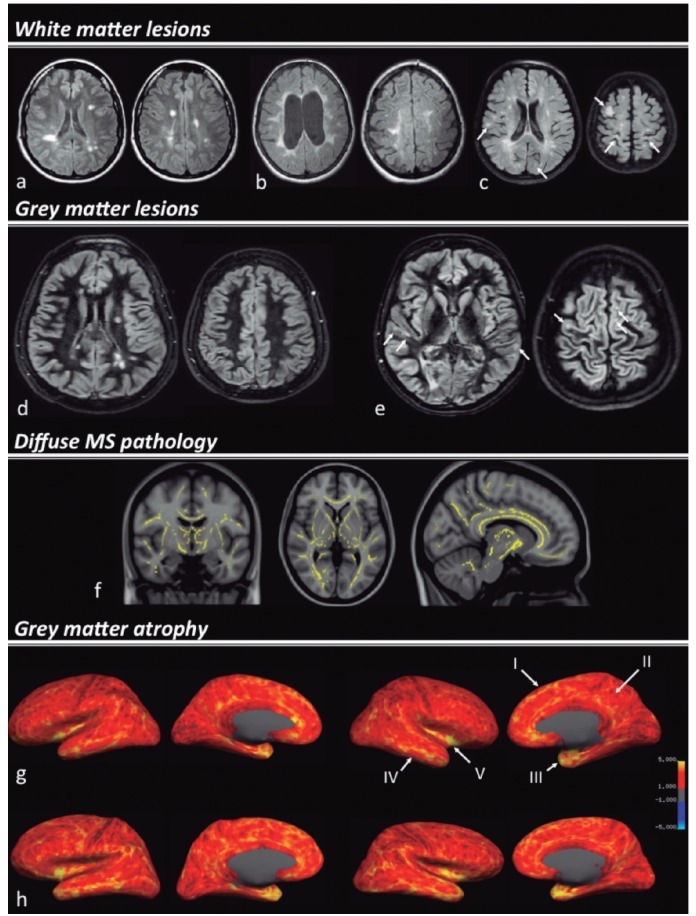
MRI metrics associated to brain damage and MS-related cognitive impairment. Examples of the multiple facets of MS pathology, white matter [A, B and C), grey matter lesions [D and E], diffuse MS pathology [F] and cortical grey matter atrophy [G and H] are demonstrated in the picture. White matter lesions are assorted in MS patients, with different distributions, according to the cognitive status. A cognitively preserved RRMS patient shows a lower lesion load, with predominance of centrally distributed WM lesions [A]; a SPMS patient [B] with mild cognitive impairment presents a higher lesion load, with confluent lesions, some of them reaching juxta-cortical locations; the more cognitively impaired subject [C], although this patient presents a lower overall lesion load than patient "b", the image shows an increased rate of (juxta)cortical lesions. Double inversion recovery (DIR) images [D and E] are sensible to detect cortical lesions. A cognitively impaired MS patient [E] has a significant higher number of cortical lesions (white arrows) than a cognitively preserved patient [D]. Fractional anisotropy differences between cognitively impaired and cognitively preserved MS patients [F - reproduced from Hulst et al.^77^, with permission of Wolters Kluwer). The brain areas in yellow display reduced fractional anisotropy in cognitively impaired patients compared to cognitively preserved patients. Cortical thickness maps in a cognitively impaired MS patient [G] and a healthy control [H]. The brain images in the left represent the measurements in the lateral and medial surfaces of the left hemisphere; the brain images in the right represent the surfaces of the right hemisphere (the cortical thickness is represented in a red to yellow scale). The MS patient shows reduced cortical thickness in several associative cortical areas in both hemispheres, compared to the healthy subject, remarkably in the superior frontal gyrus (I), posterior cingulate (II), temporal pole (III), temporal lobe (IV) and insula (V).
